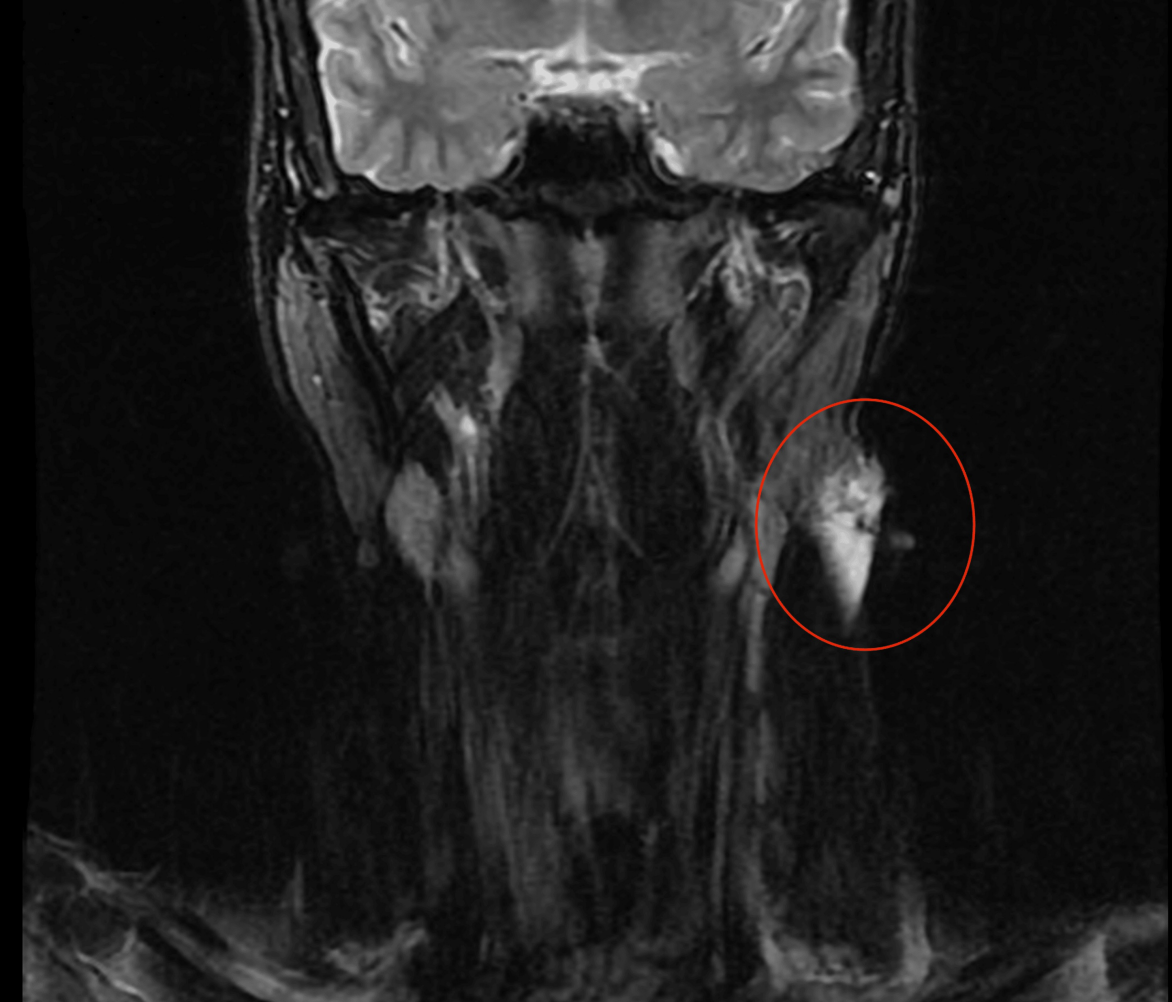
Coronal T2-weighted MRI showing stability in lesion size and morphology over time (lesion circled in red).
